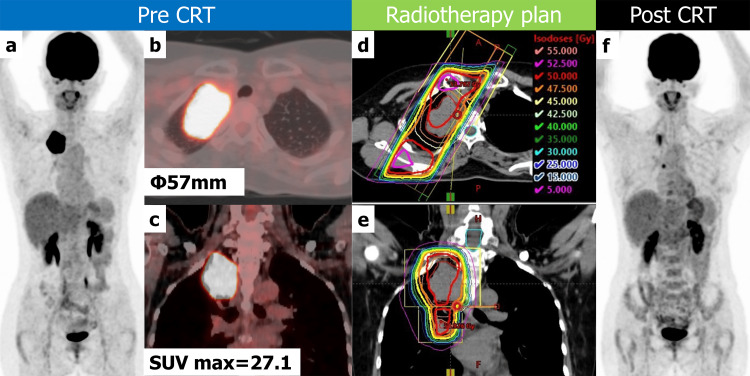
Pre- and post-therapy 18F-FDG PET/CT and treatment planning CT a-c: pre-treatment ^18^F-FDG PET/CT: (a) maximum intensity projection (MIP); (b) axial fusion image of PET/CT with pulmonary window; (c) coronal fusion image of PET/CT with soft tissue window. d-e: radiation planning CT. f: post-treatment ^18^F-FDG PET/CT MIP image. In the pre-treatment positron emission tomography (PET)/CT, there is a 57 mm tumor in the right upper lobe S1 adjacent to the mediastinum, showing an ^18^F-FDG uptake with a maximum standardized value of 27.1 (a-c). In the treatment planning CT, bold circles indicate isodose lines. Thin red, orange, yellow, and cyan circles indicate the gross tumor volume (GTV), clinical target volume (CTV), planning target volume (PTV), and spinal canal, respectively (d, e). In the post-treatment ^18^F-FDG PET/CT, a reduction in the lesion can be observed. The uptake near the vertebrae is non-specific, possibly due to degenerative changes.
